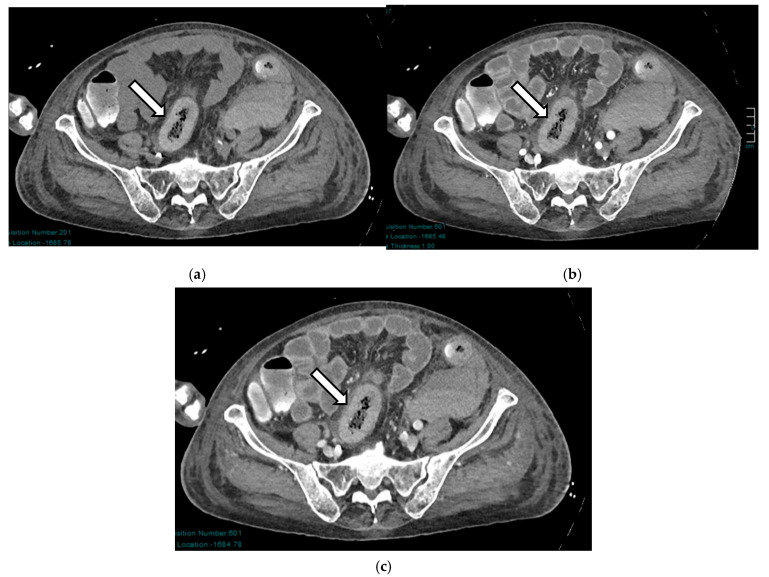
NOMI in a 78-year-old man: on axial pre- and post-contrast CT images, arrows show thickened bowel wall with mucosal hyperenhancing at unenhanced scans (a); in post-contrast CT scans, bowel walls show no enhancing (b,c).
